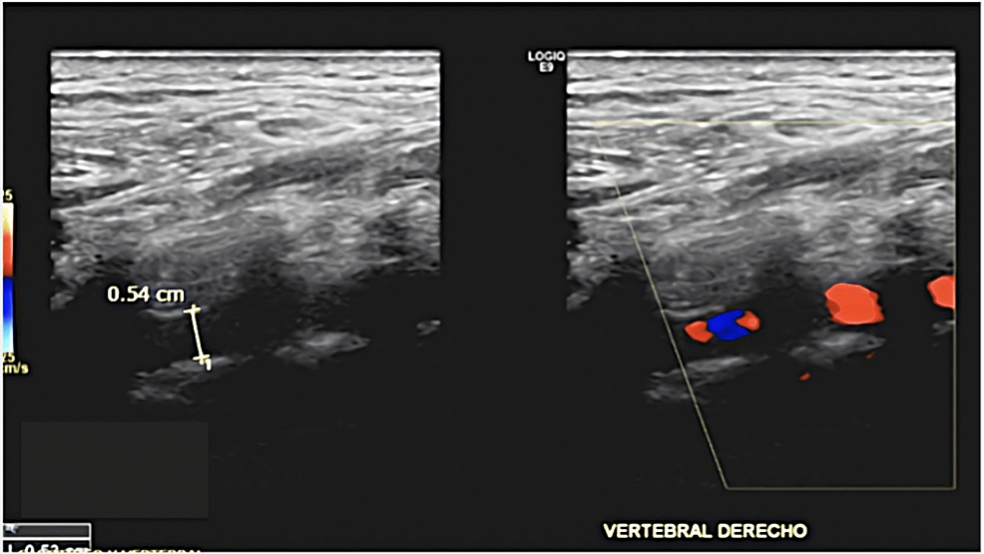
Vertebral Artery Doppler. Increased diameter of the right vertebral artery.
